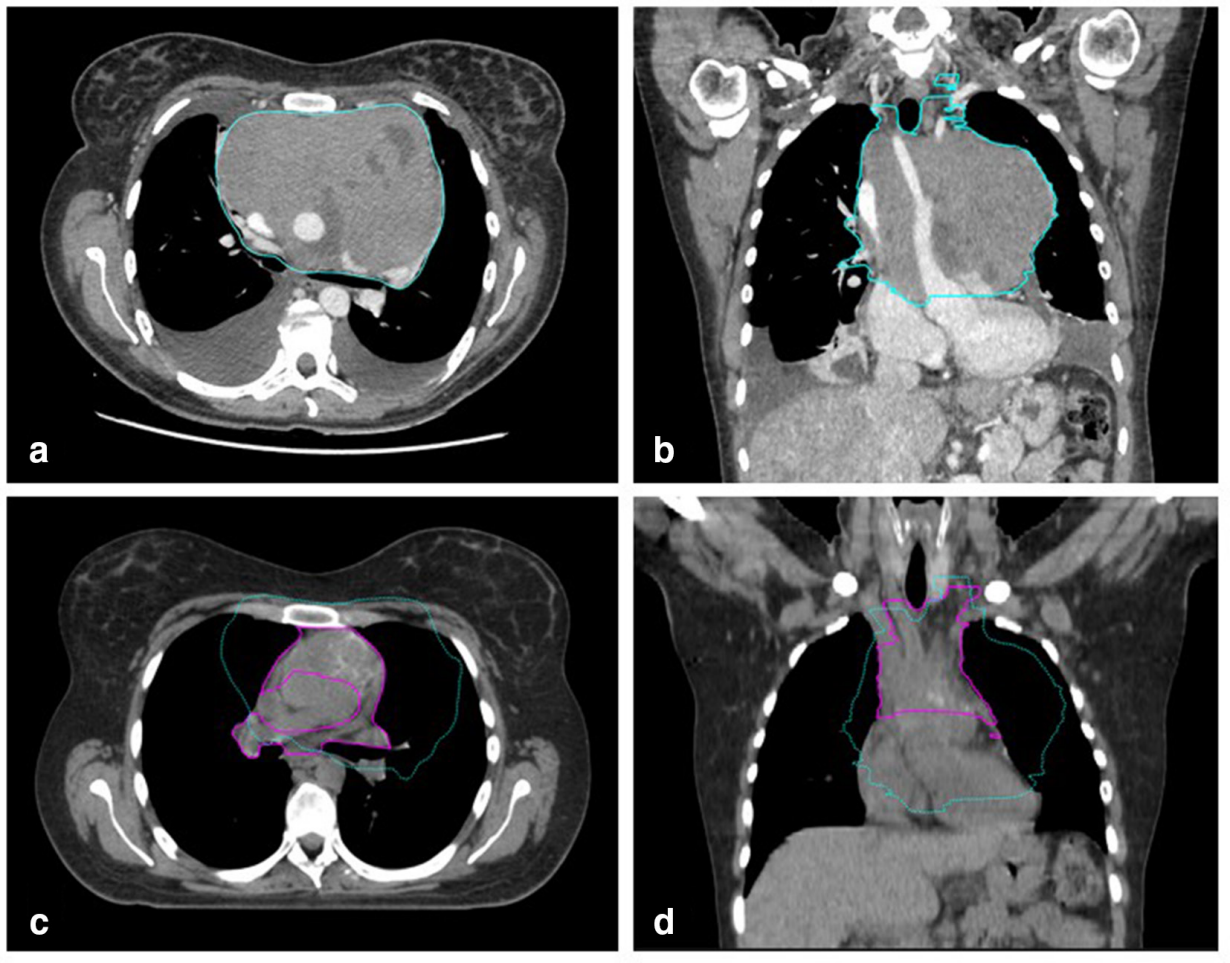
Contouring process according to the ISRT principles. Pre-chemotherapy GTV is contoured on the baseline radiologic exams (blue volume in a and b). Planning CT scan is then co-registered with pre-chemotherapy exams in order to translate the GTV volume (blue volume in c and d). The same GTV volume then needs to be modified to generate the CTV (pink volume in c and d), in order to exclude healthy organs such as muscles, vessels lungs and heart that were clearly uninvolved at diagnosis.
